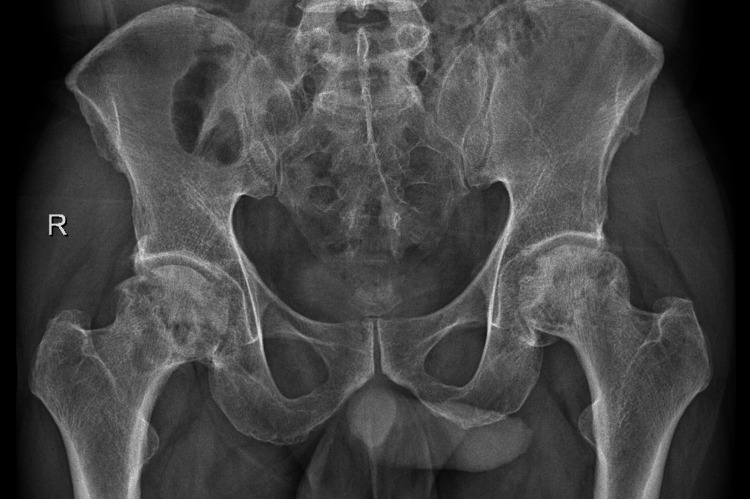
A plain radiograph of the pelvis and bilateral hip joints was obtained, which depicted mild sclerosis, cystic changes, and changes in osteonecrosis
